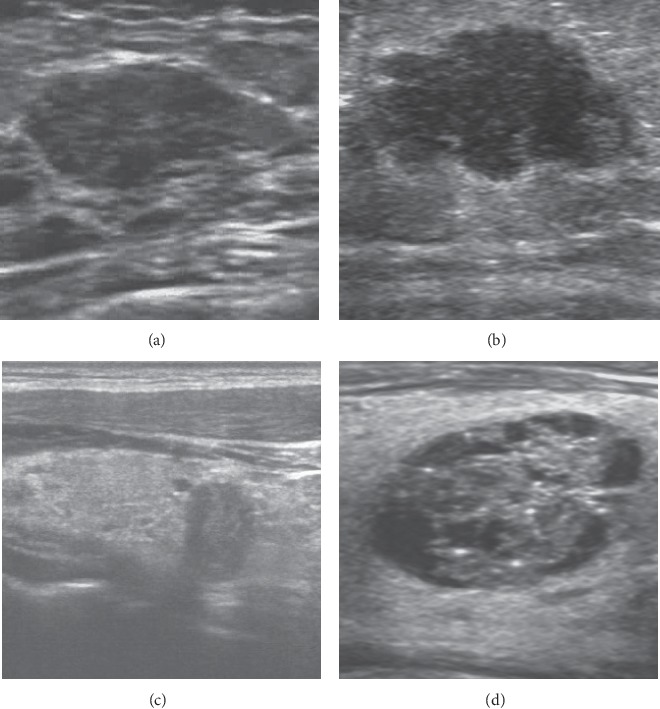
Diagnosis of thyroid and breast nodules with benign or malignant tendency by ultrasound guidelines and CNN. (a) Fibroadenoma. The nodule was classified as BI-RADS 3, while the diagnosis of CNN was benign tendency (93.24%). (b) Invasive breast carcinoma. The nodule was classified as BI-RADS 4, while the diagnosis of CNN was malignant tendency (100%). (c) Papillary thyroid carcinoma. The nodule was classified as TR5, while the diagnosis of CNN was malignant tendency (99.39%). (d) Nodular goiter. The nodule was classified as TR2, while the diagnosis of CNN was benign tendency (100%).
